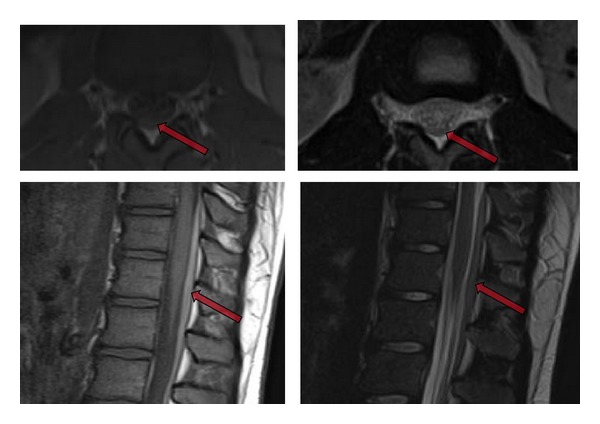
Lumbar spine MRI [Axial and sagittal T1-weighted (left top and bottom); axial and sagittal T2-weighted (right top and bottom) images] demonstrates dorsal subdural hematoma (arrows) extending from approximately T11/12 to L4 levels.
